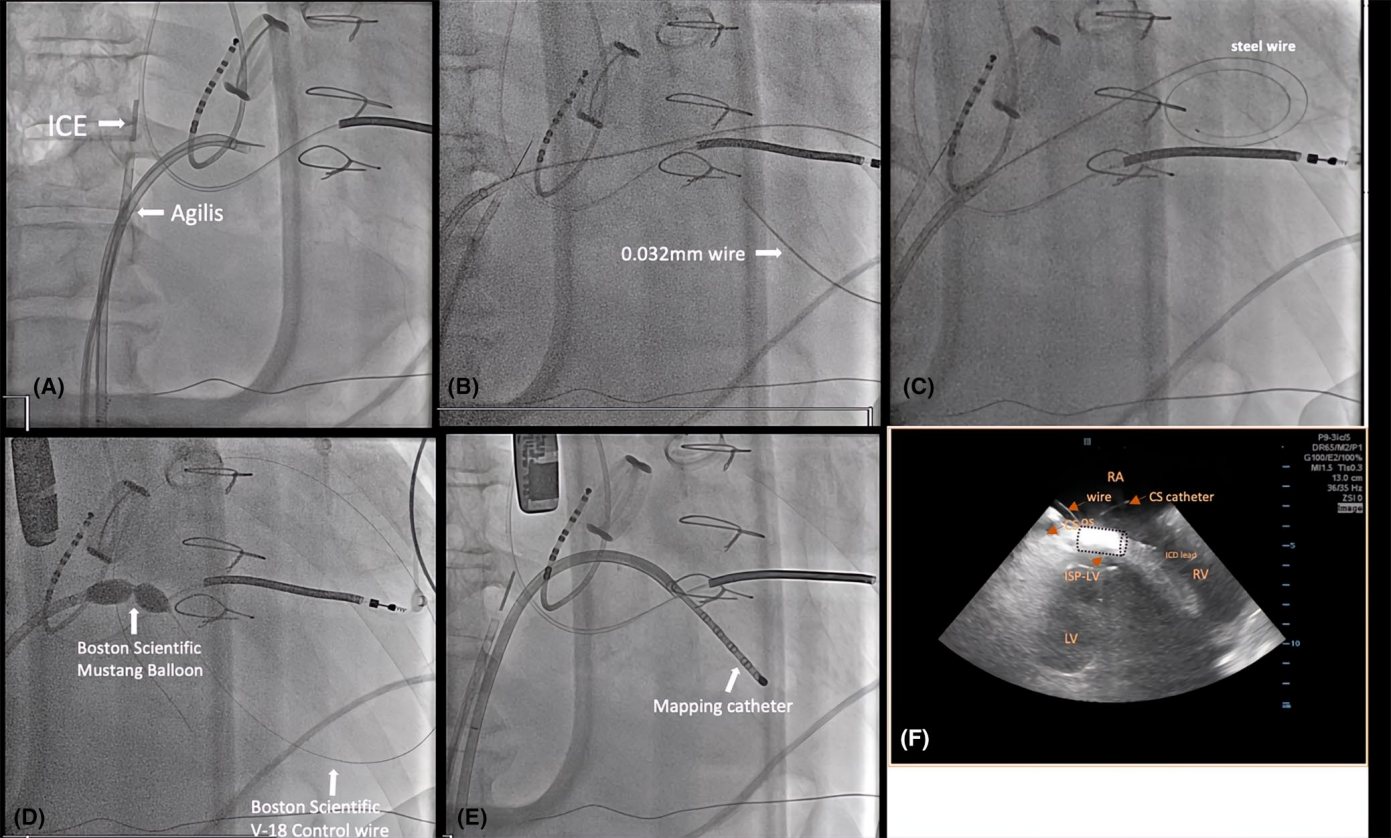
Steps in Right atrium to Left ventricle puncture. (A) Puncture of the inferior RA septum using cautery; (B) Once puncture was made, the 0.032 mm wire was inserted through the puncture site and into the LV; (C) The 0.032 wire was replaced with steel wire; (D) The steel wire was exchanged with Boston Scientific V‐18 Control wire. The ST‐01 catheter was changed with Boston Scientific Mustang Balloon and placed into the puncture site and several dilatations were done; (E) Balloon catheter and control wire removed; Mapping catheter placed inside the LV; (F) ICE at inferolateral RA. The 0.032 wire was positioned at inferior RA septum and visualized as it punctured into the LV. ICE, intracardiac echocardiography; LV, left ventricle: RA, right atrium.
